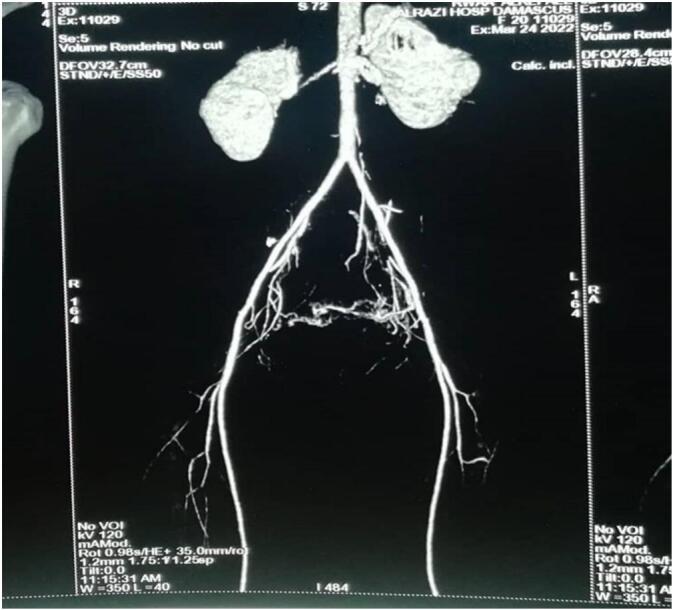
Computed tomography angiography shows absence of the past fistulas.
